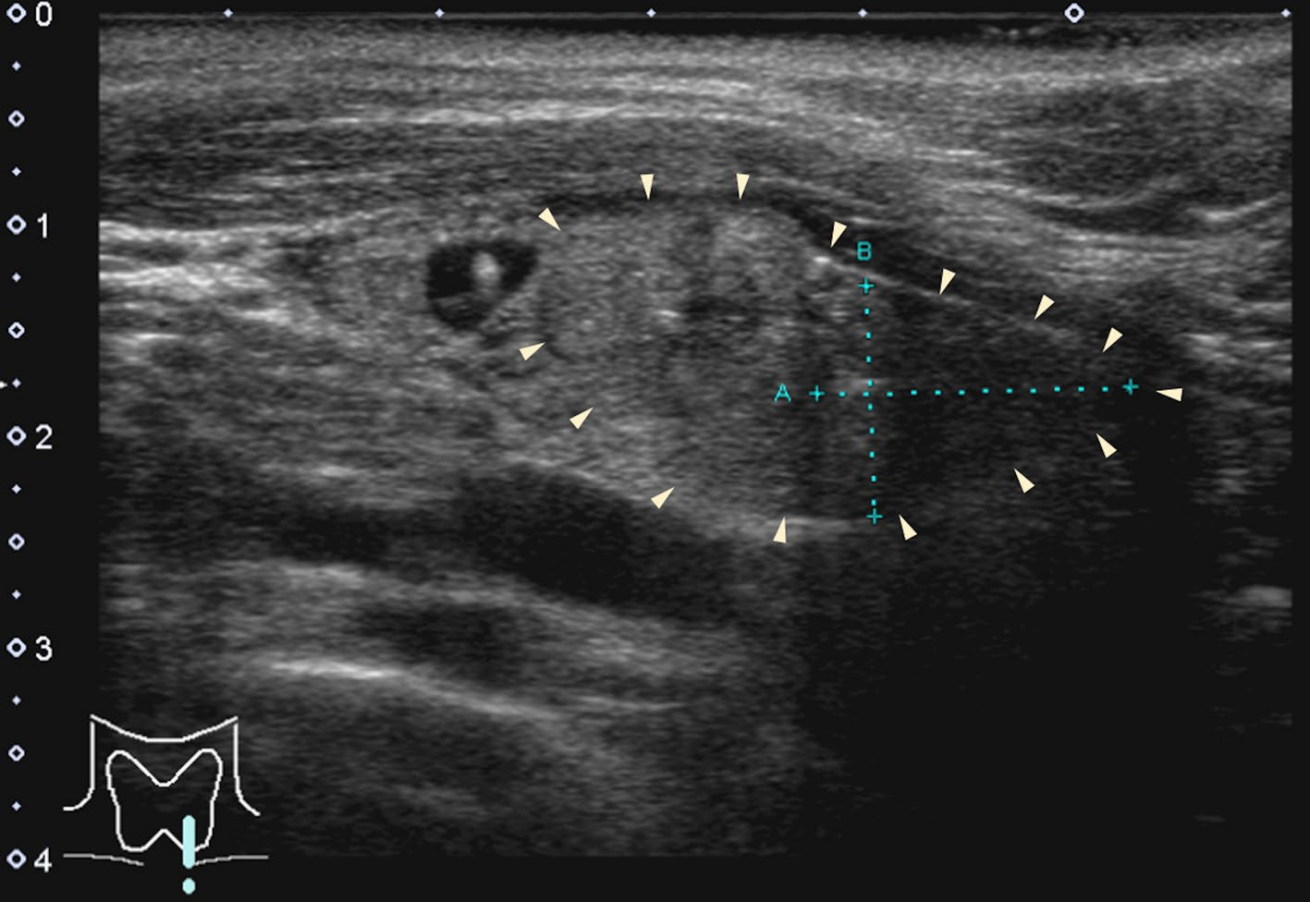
A parathyroid adenoma with heterogeneous echogenicity (Group 2). Triangular arrows indicate the margins of the tumor. On ultrasound examination, only a hypoechoic area was recognized as a tumor
